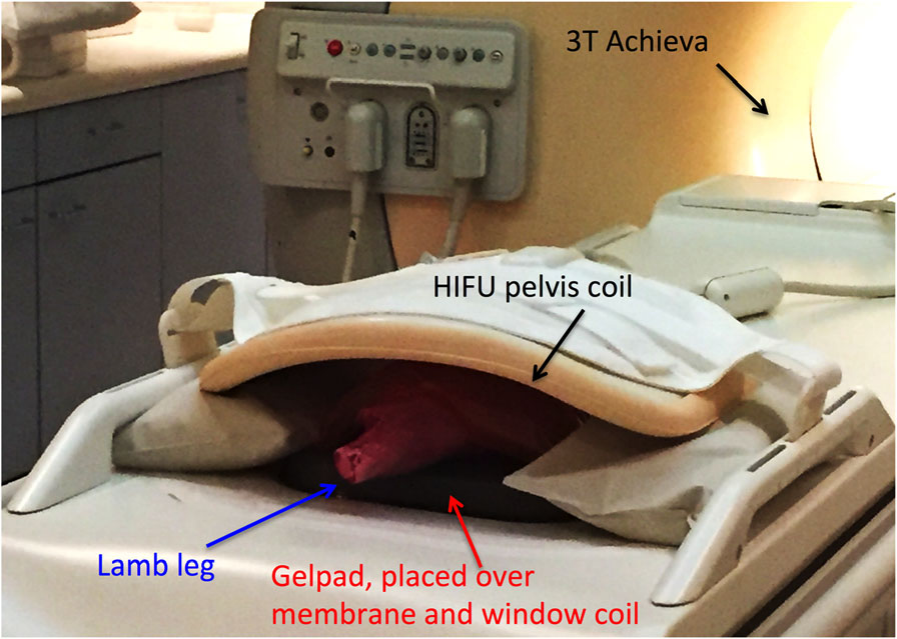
A lamb leg sample shown mounted for sonication in close acoustic contact with a dampened Aquaflex gelpad. The gelpad was acoustically coupled to the membrane covering the high-intensity focused ultrasound (HIFU) window coil using de-gassed water. The mobile, 256-element, 14 cm radius-of-curvature, phased-array ultrasound equipment is located beneath this membrane. The lamb leg sample was secured in place using the HIFU pelvis coil. The pelvis and window coil were operated in dual coil mode for imaging
